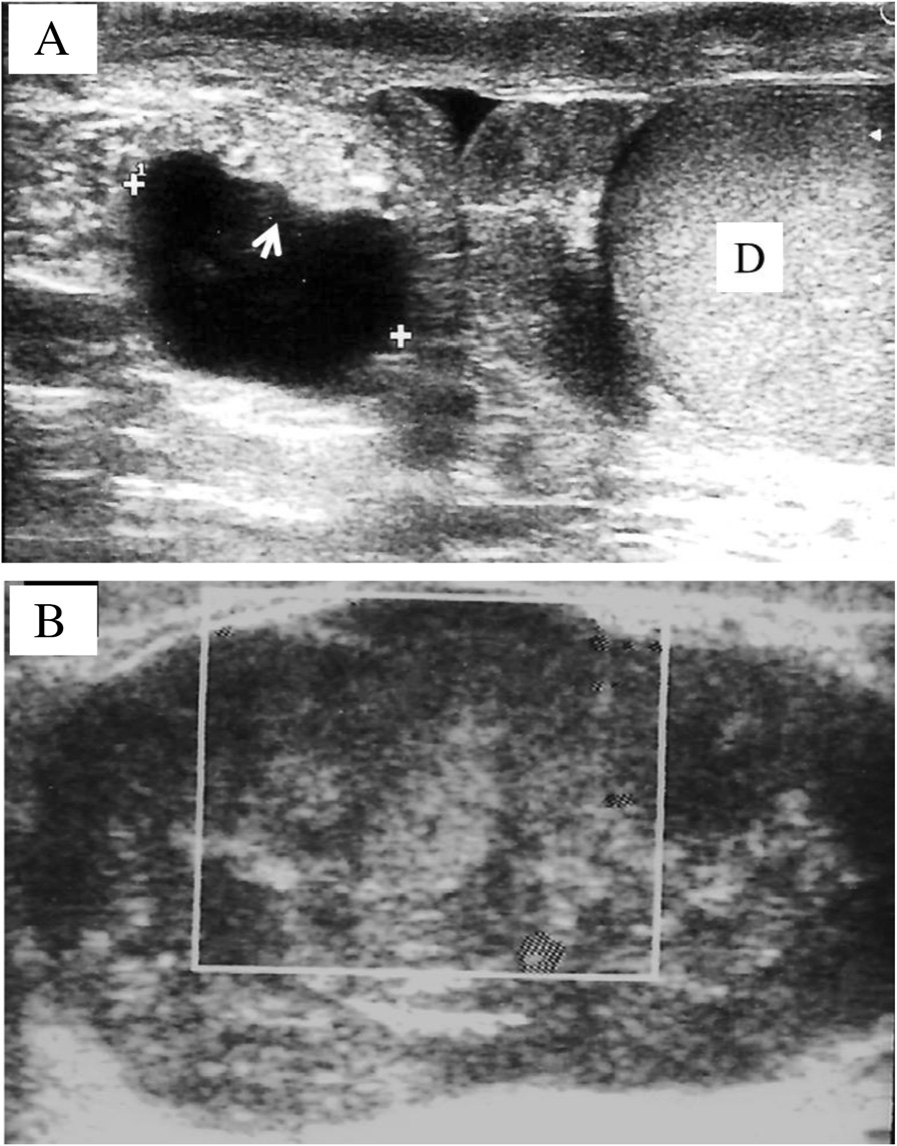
Scrotal ultrasound. A. 2-cm lesion with cystic appearance and intracystic material (Arrow), close to the head of the epidydimis, and separated from the didimis (D). B. Preoperative scrotal untrasound shows a heterogeneous mass.
